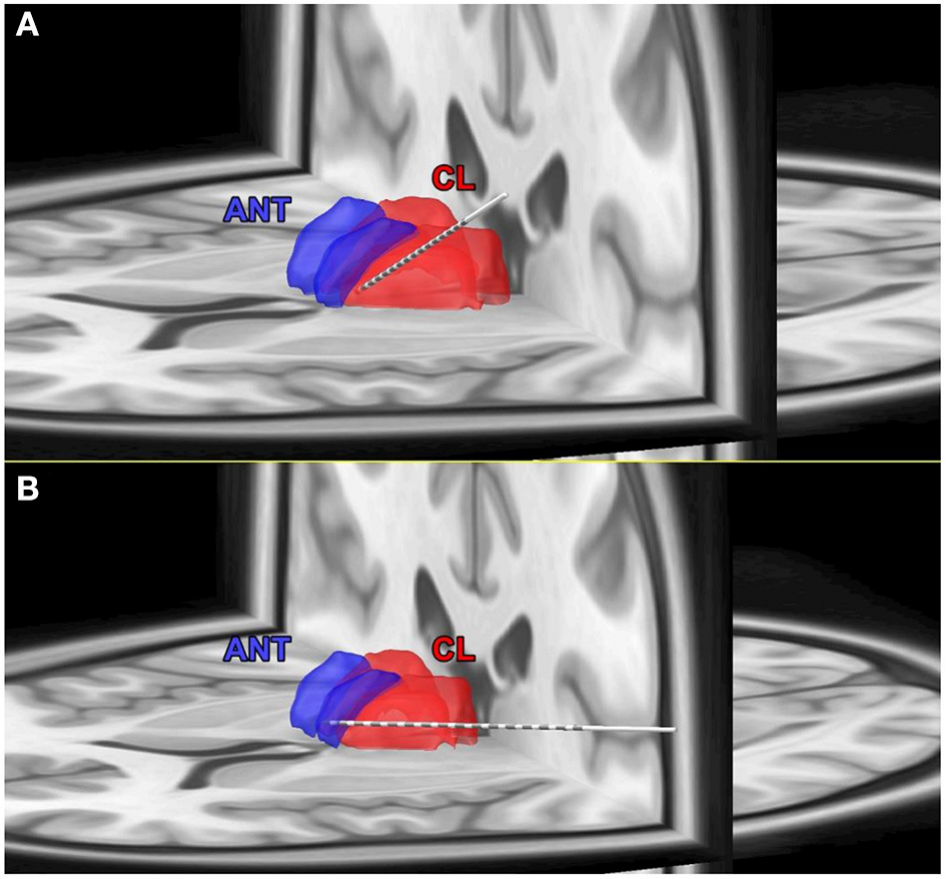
(A) Coregistration of post-implant CT scan on pre-implant MRI and Morel's thalamic atlas to determine the exact localization of thalamic targets. (A) An example of the electrode localized to the central thalamic nucleus (CL). (B) An example of the electrode localized to the anterior thalamic nucleus (ANT).
